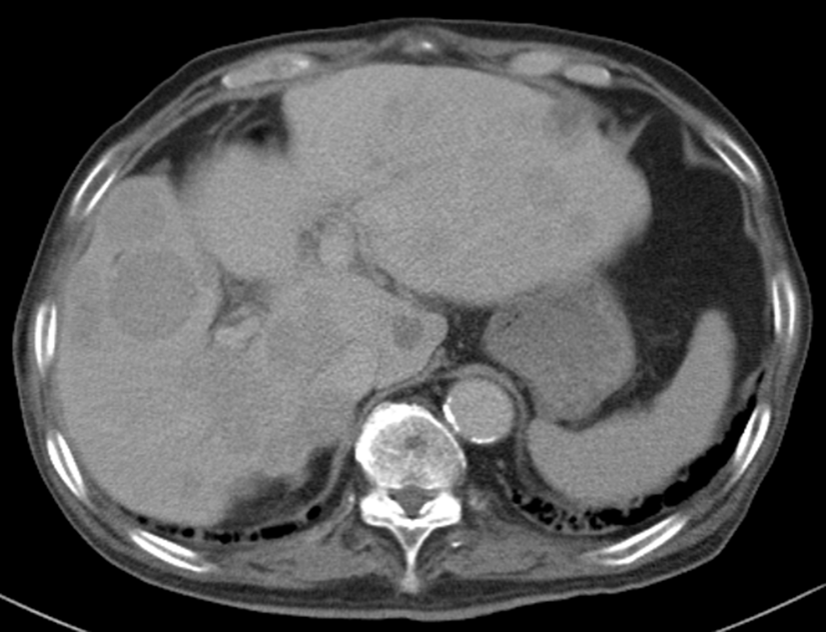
Computed tomography. Post-operative 2 years computed tomography at portal phase showing multicentric recurrence of hepatocellular carcinoma in the liver.
